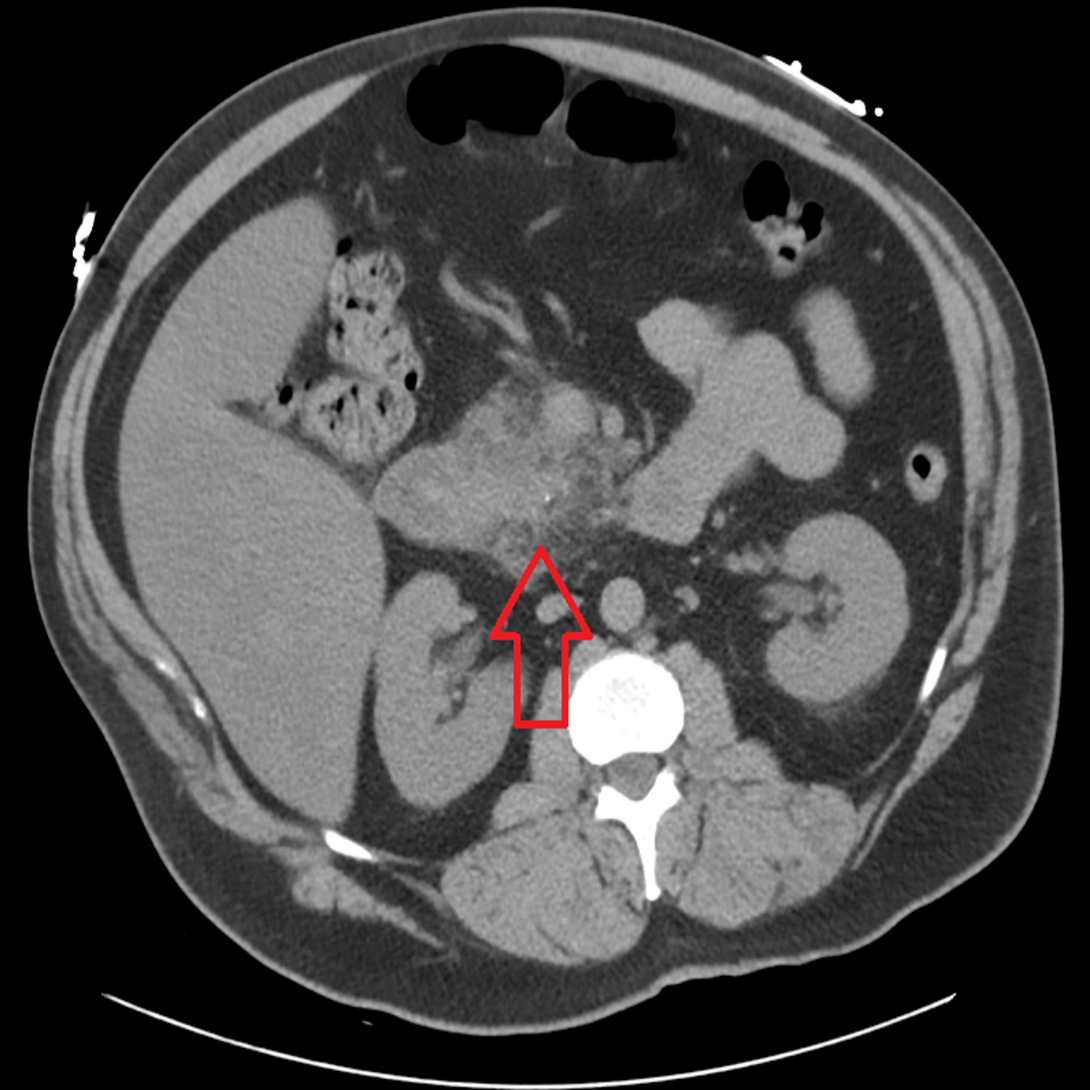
CT scan showing an inflammatory change located within the pancreatic head consistent with pancreatitis.
